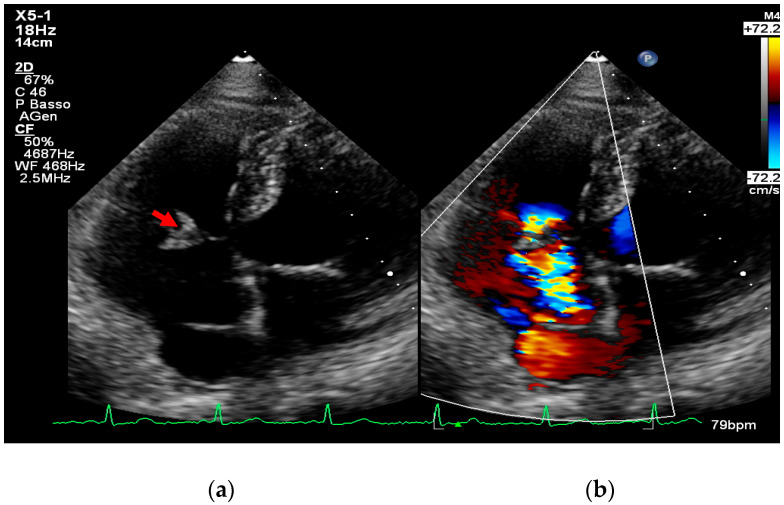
(a,b). Transthoracic echocardiogram showing tricuspid valve vegetation (arrow, a) and severe regurgitation (b) caused by infectious damage to the septal leaflet. This is an original, de-identified clinical image from our institution. Patient consent for publication was obtained.
